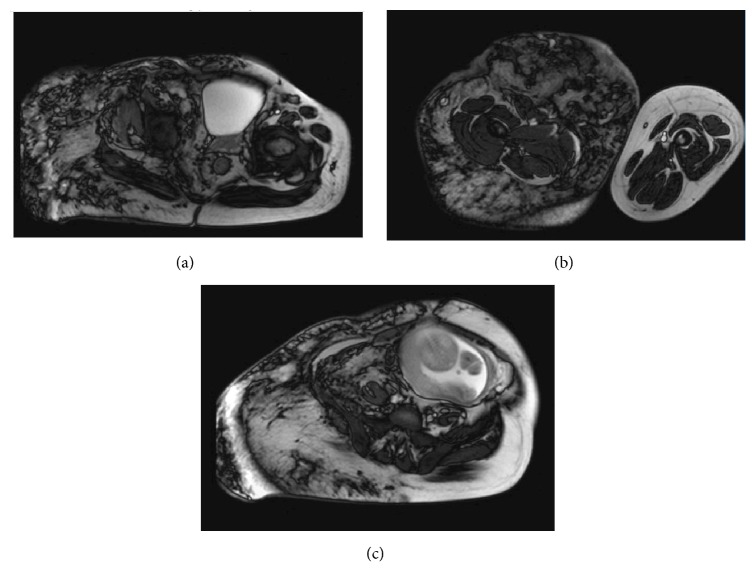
MRI images depicting. (a) Marked hemihypertrophy of the subcutaneous soft tissues on the right side at the level of the lower abdomen. (b) Marked hemihypertrophy of the subcutaneous soft tissues on the right thigh as compared to the left (normal). (c) Numerous venous channels were noted to cross at the potential epidural injection sites from L1 to L5. The thickness from the skin to the thecal space was 9.4 cm.
